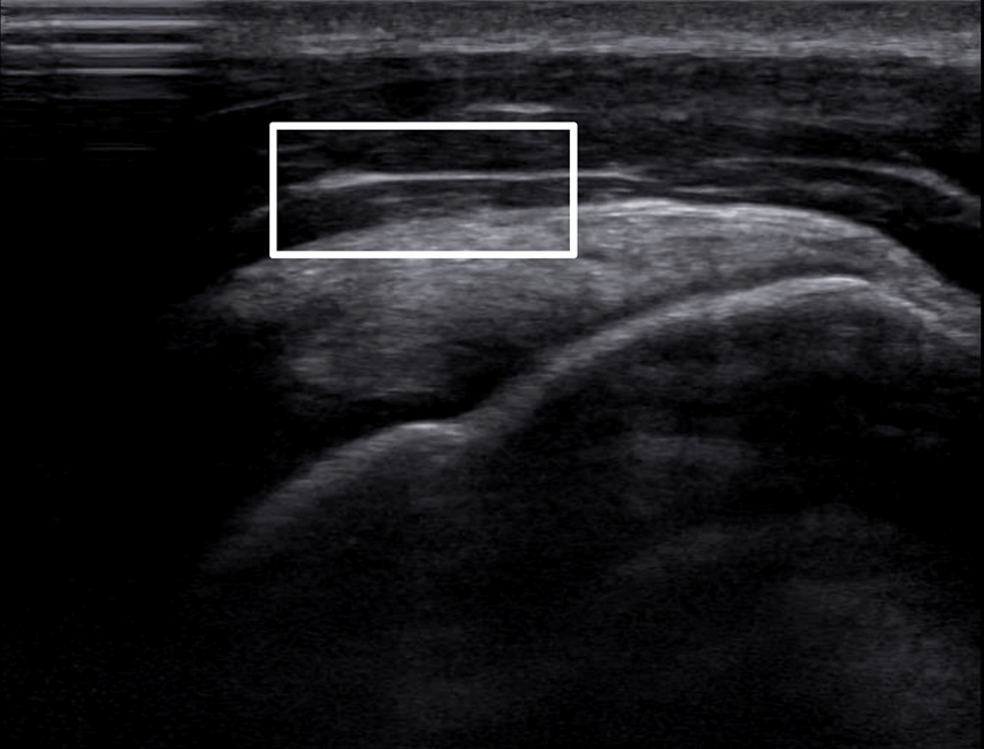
First post-treatment ultrasound view of the right anterior supraspinatus
